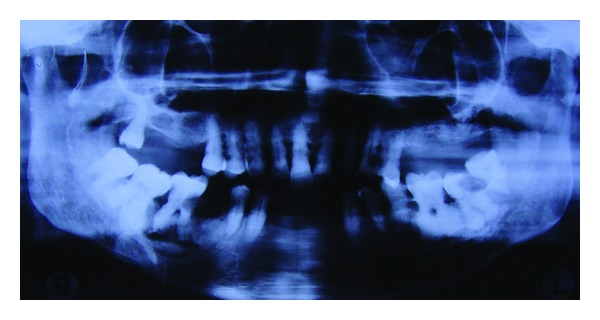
Extraoral imaging: conventional orthopantomogram confirming the presence of bulbous roots with irregular outline, bilaterally seen in the second premolars and first and second molars and revealing multiple missing teeth with generalized interdental bone loss.
